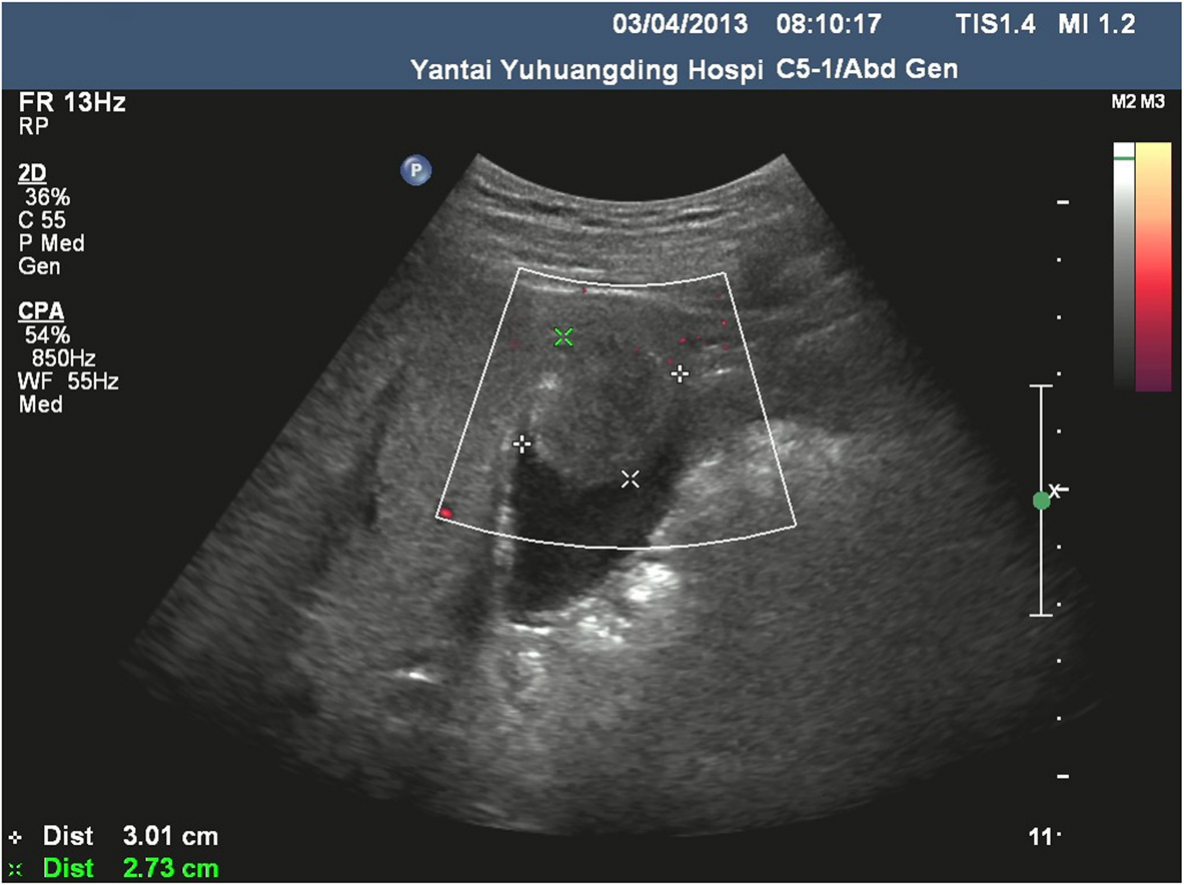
Ultrasonography B (Ultra-B) showed increasing volume of gallbladder, thicker wall and tumor.
